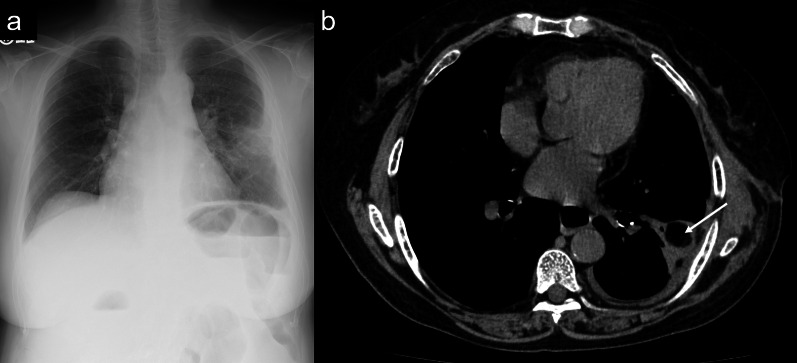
Chest radiography and computed tomography (CT) after discharge. a Chest radiograph showing decreased transparency in the middle lung field because of the implanted free pericardial fat (FPF); however, other parts of the lungs were well expanded. b Chest CT image showing the implanted FPF within the abscess cavity as a low-density area (white arrow)
